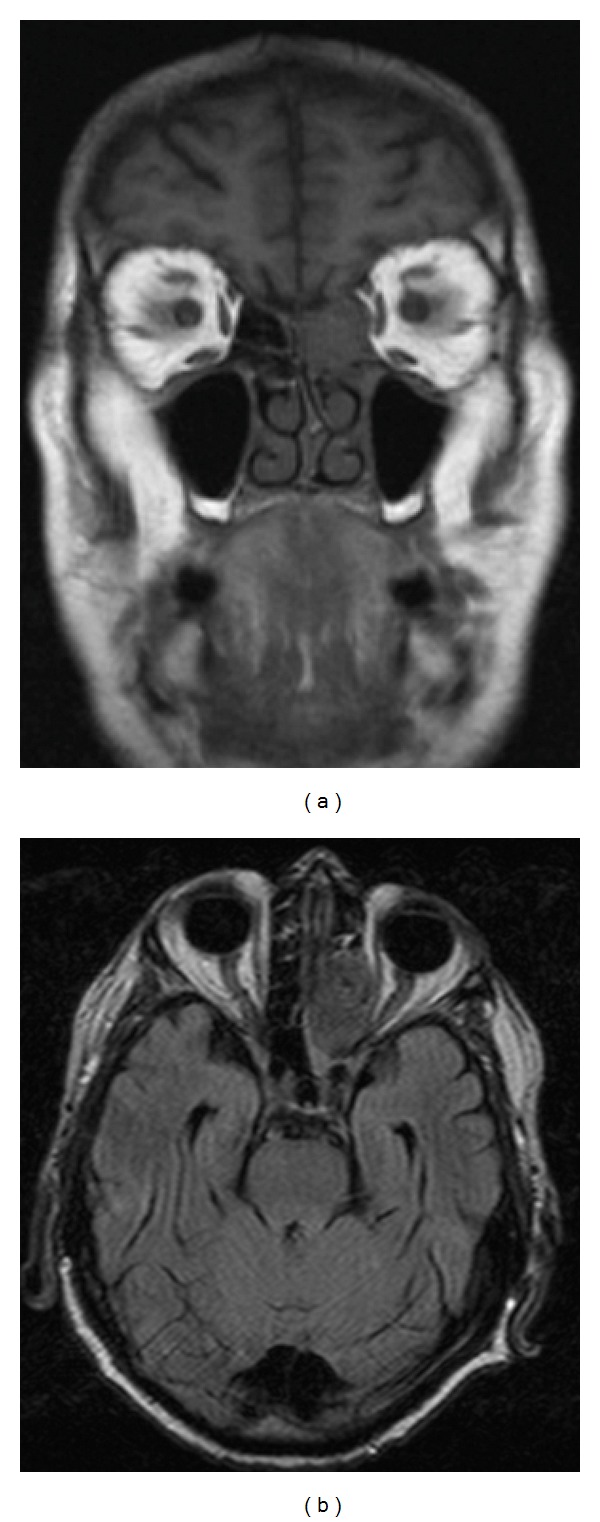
MRI scan shows an abnormal soft tissue lesion within the left paranasal sinuses centered on the ethmoid air cells. Note the distortion of the medial wall of the orbit and narrowing of the orbital apex causing distortion and compression of the left optic nerve.
